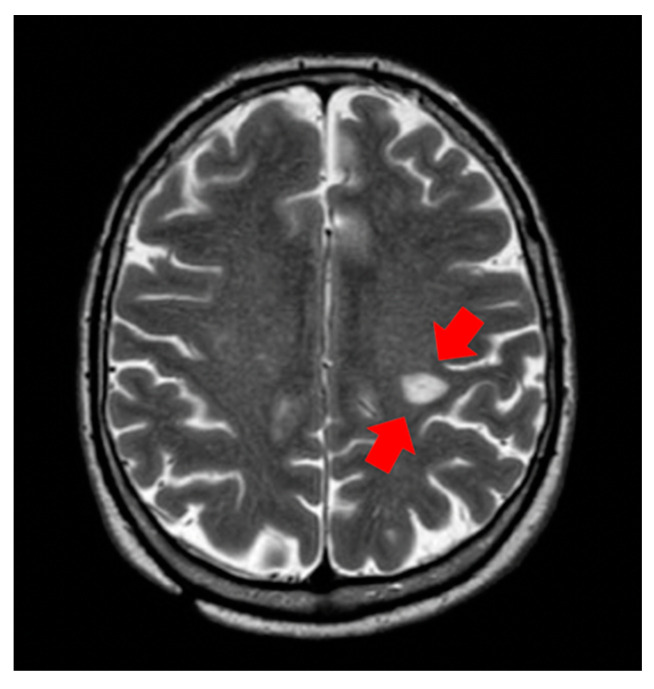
Second brain magnetic resonance imaging performed after discontinuation of sunitinib and steroid therapy.
